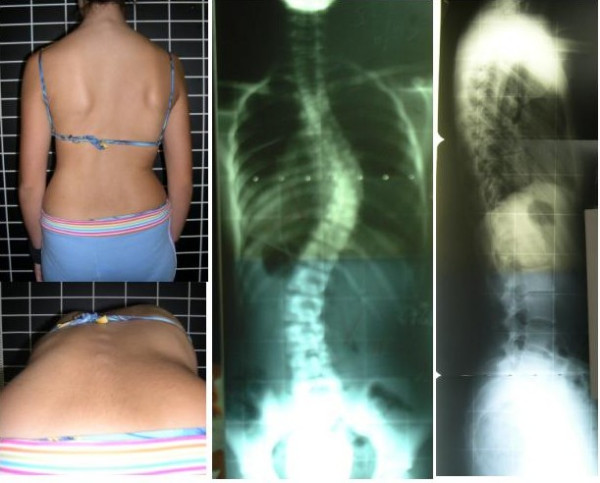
Patient information included dorsal and forward bending photographs, as well as AP and latero-lateral radiographs.
